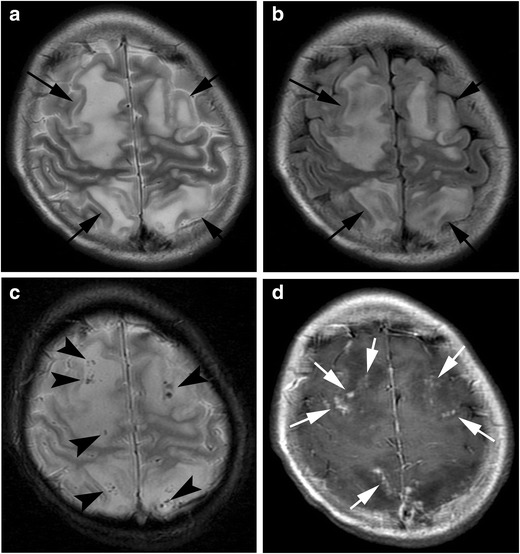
Haemophagocytic lymphohistiocytosis. A 14-year-old girl with myelodysplastic syndrome presented with seizure. a A T2-weighted image shows patchy hyperintensities with swelling in the frontal and parietal lobes (*arrows*). b FLAIR image shows hyperintensity of these lesions (*arrows*). c T2*-weighted image shows a number of microhaemorrhages in these lesions (*arrowheads*). d Post-contrast image shows nodular enhancement along the leptmeninx (*white arrows*)
